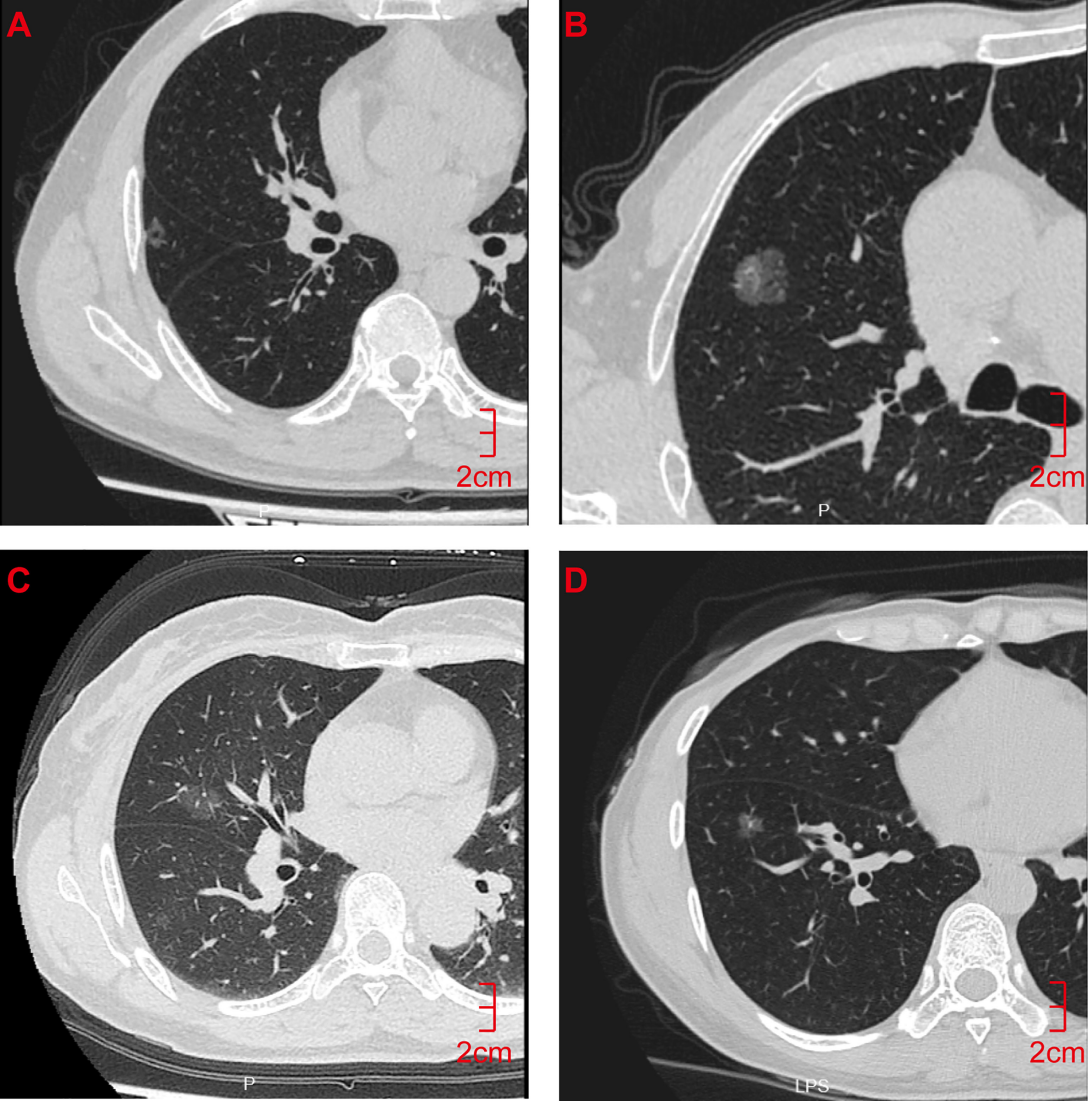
CT Findings of representative IAC and MIA nodules. (A) M/55y, pGGN of the right upper lobe with a long diameter of 1.2 cm, pathological diagnosis showed that the lesion was IAC. (B) M/32y, mGGN of the right upper lobe with a long diameter of 1.5 cm, pathological diagnosis showed that the lesion was IAC. (C) F/62y, pGGN with a long diameter of 1.2 cm in the right middle lobe, pathological diagnosis showed that the lesion was MIA. (D) F/55y, pGGN with a long diameter of 1.0 cm in the right lower lobe, pathological diagnosis showed that the lesion was MIA. CT, computed tomography; pGGN, pure ground-glass nodule; mGGN, mixed ground-glass nodules; IAC, invasive adenocarcinoma; MIA, minimally invasive adenocarcinoma.
